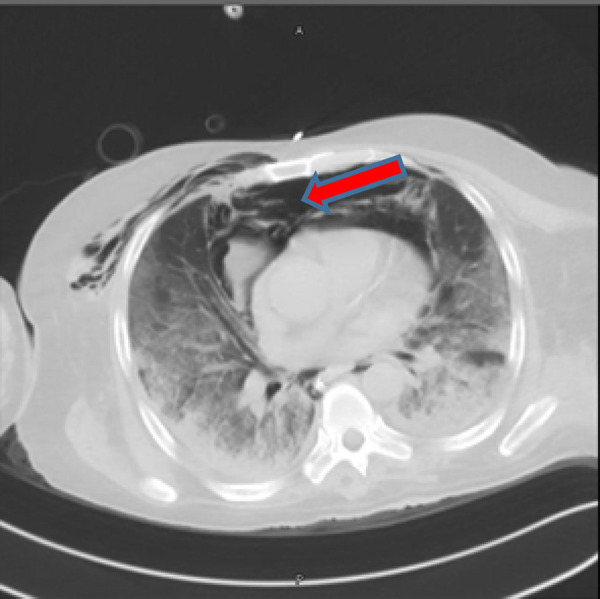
Computed tomography of the chest showing tension pneumomediastinum
